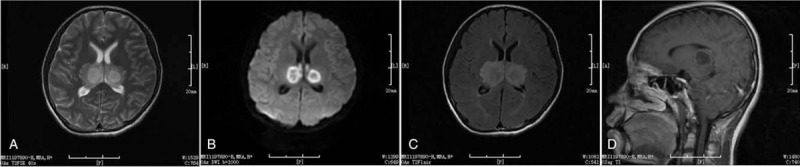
Brain magnetic resonance imaging of a 15-year-old female. The scans showed multiple symmetrical brain lesions (slightly ring enhancing) in bilateral dorsal areas of the thalami, dorsal caps of the pontine, and bilateral cerebellar hemispheres. (A) T2. (B) Diffusion-weighted imaging. (C) T2 flair. (D) T1 enhanced.
